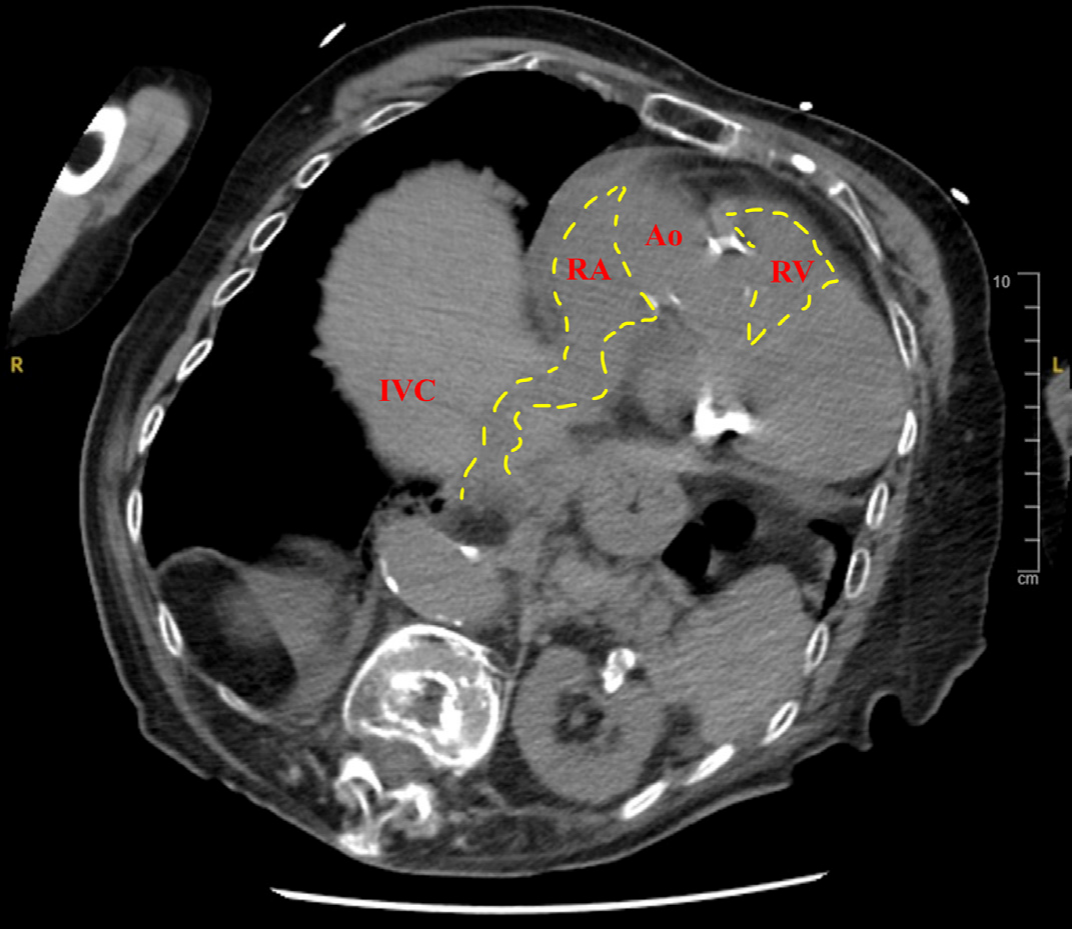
Computed Tomography Computed tomography image showing anterior elongation of the inferior vena cava (IVC)–right atrium (RA) junction (yellow dashed lines) caused by the kyphosis. Ao = aorta; RV = right ventricle.
